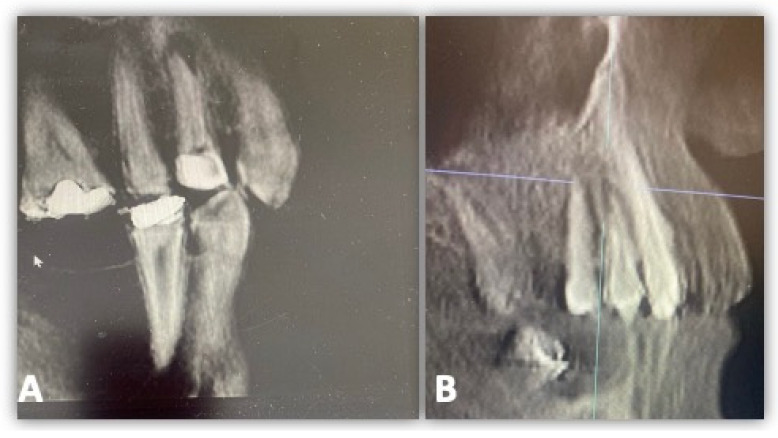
Radiographical CBCT images of cases included in this study: (A) tooth #5 with AAP, (B) another case tooth #5 with SAP.
